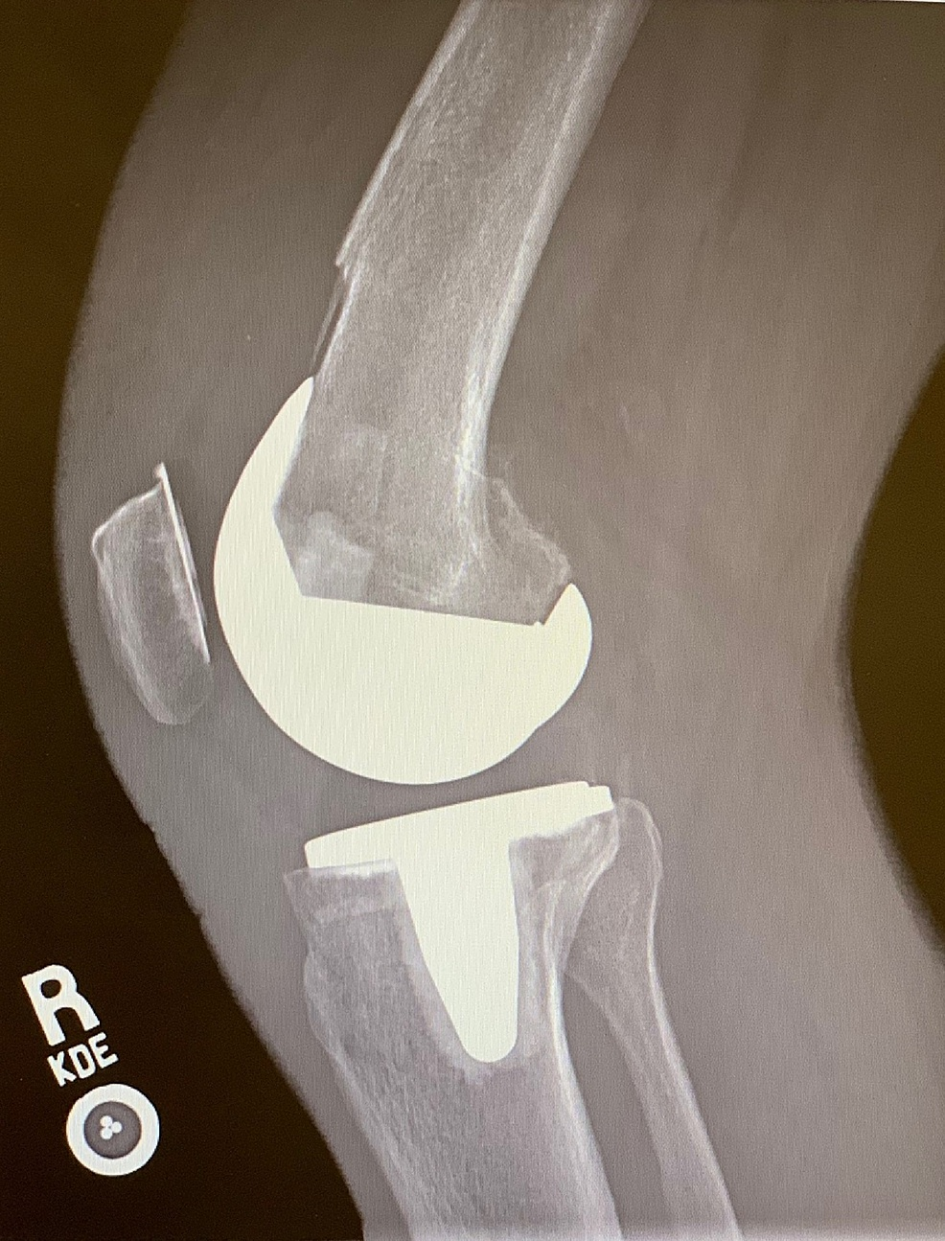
Lateral preoperative radiograph of the right knee demonstrating no obvious signs of aseptic loosening of the components.
